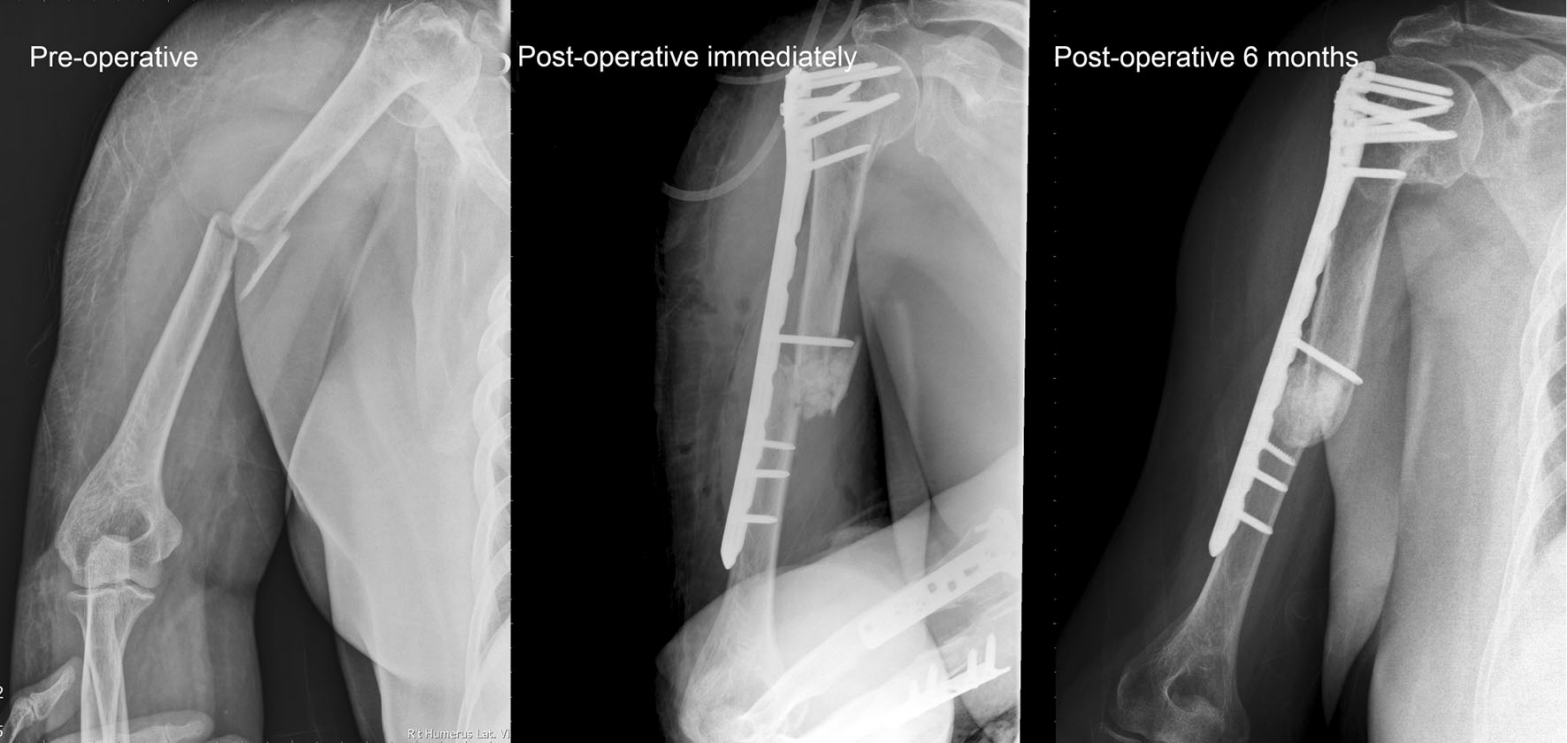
A 66-year-old women had right humeral shaft and neck fracture. She underwent open reduction internal fixation via a minimally invasive technique and Bicera™ was filled into fracture. Radiograph revealed fracture union 6 months after operation
